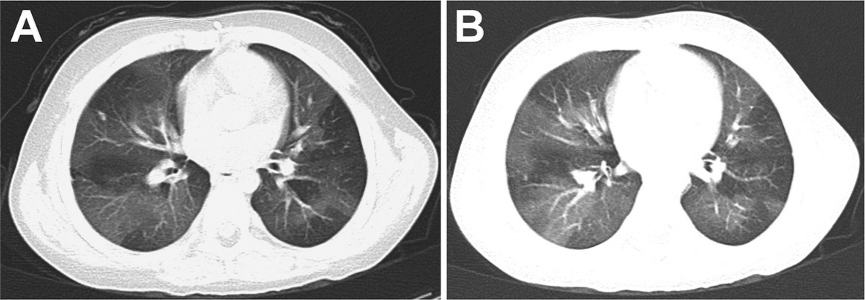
Pre-treatment (A) and post-treatment (B) HRCT scans of the lung in a 5-year-old boy. There was no significant improvement following treatment.
